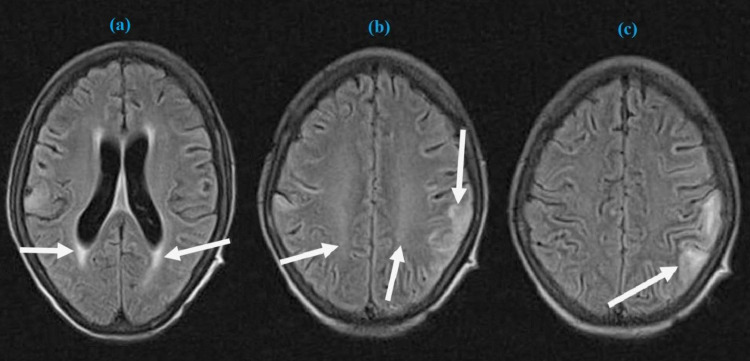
Axial images of brain MRI showing patchy areas of hyperintense signals (white arrows) in cortical and subcortical areas of temporal, parietal (a, b), and occipital lobes (c). MRI: magnetic resonance imaging.
